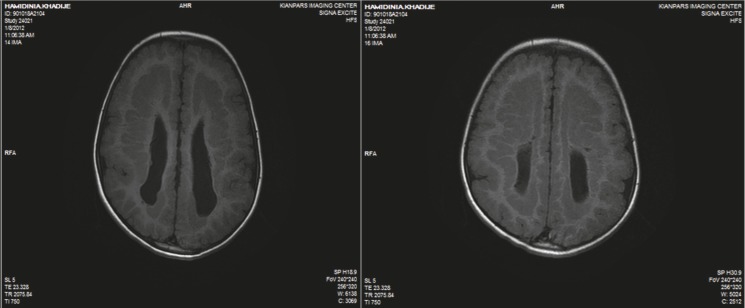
Axial T1-weighted brain MRI shows polymicrogyria, complete band heterotopia, and colpocephaly
